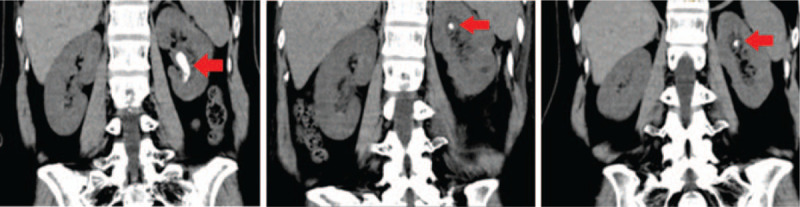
The second percutaneous nephrolithotomy computed tomography (CT) images and postoperative reexamination results. Left panel, the CT scan of the urinary system on June 10, 2020 revealed that there were more left renal calyx stones than before (April 13, 2020). Middle panel postoperative examination of the CT scan of the urinary system revealed that the left kidney stones were significantly reduced on June 16, 2020. Right panel, on August 23, 2020, the CT scan shows that there was no significant change in the residual stones with the middle panel.
